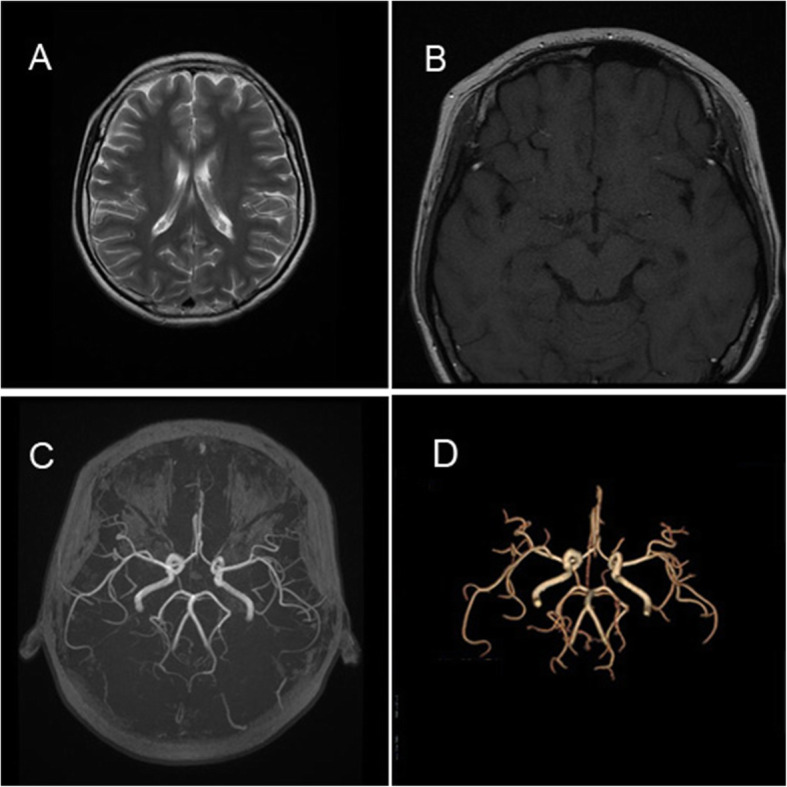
Neuroimaging. An axial cranial fast spin-echo T2-weighted (a) and T1-weighted (b) magnetic resonance imaging revealed no cerebral calcification or atrophy. Three-dimensional time-of-flight (c) and volume rendering (d) for magnetic resonance angiography demonstrates no intracranial leptomeningeal angiomatosis
